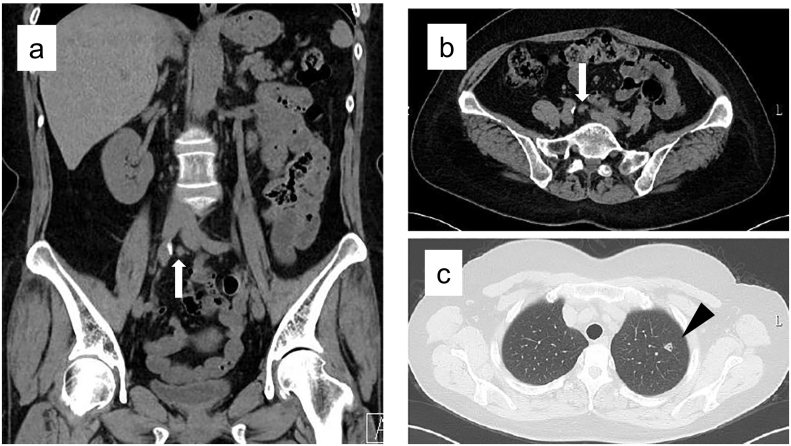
Abdominal computed tomography scan showed an 8 mm nodule (arrow) on the caudal side of the right common iliac artery (a, b), and an 8 mm nodule (arrowhead) in the left upper lobe of the lung (c).
